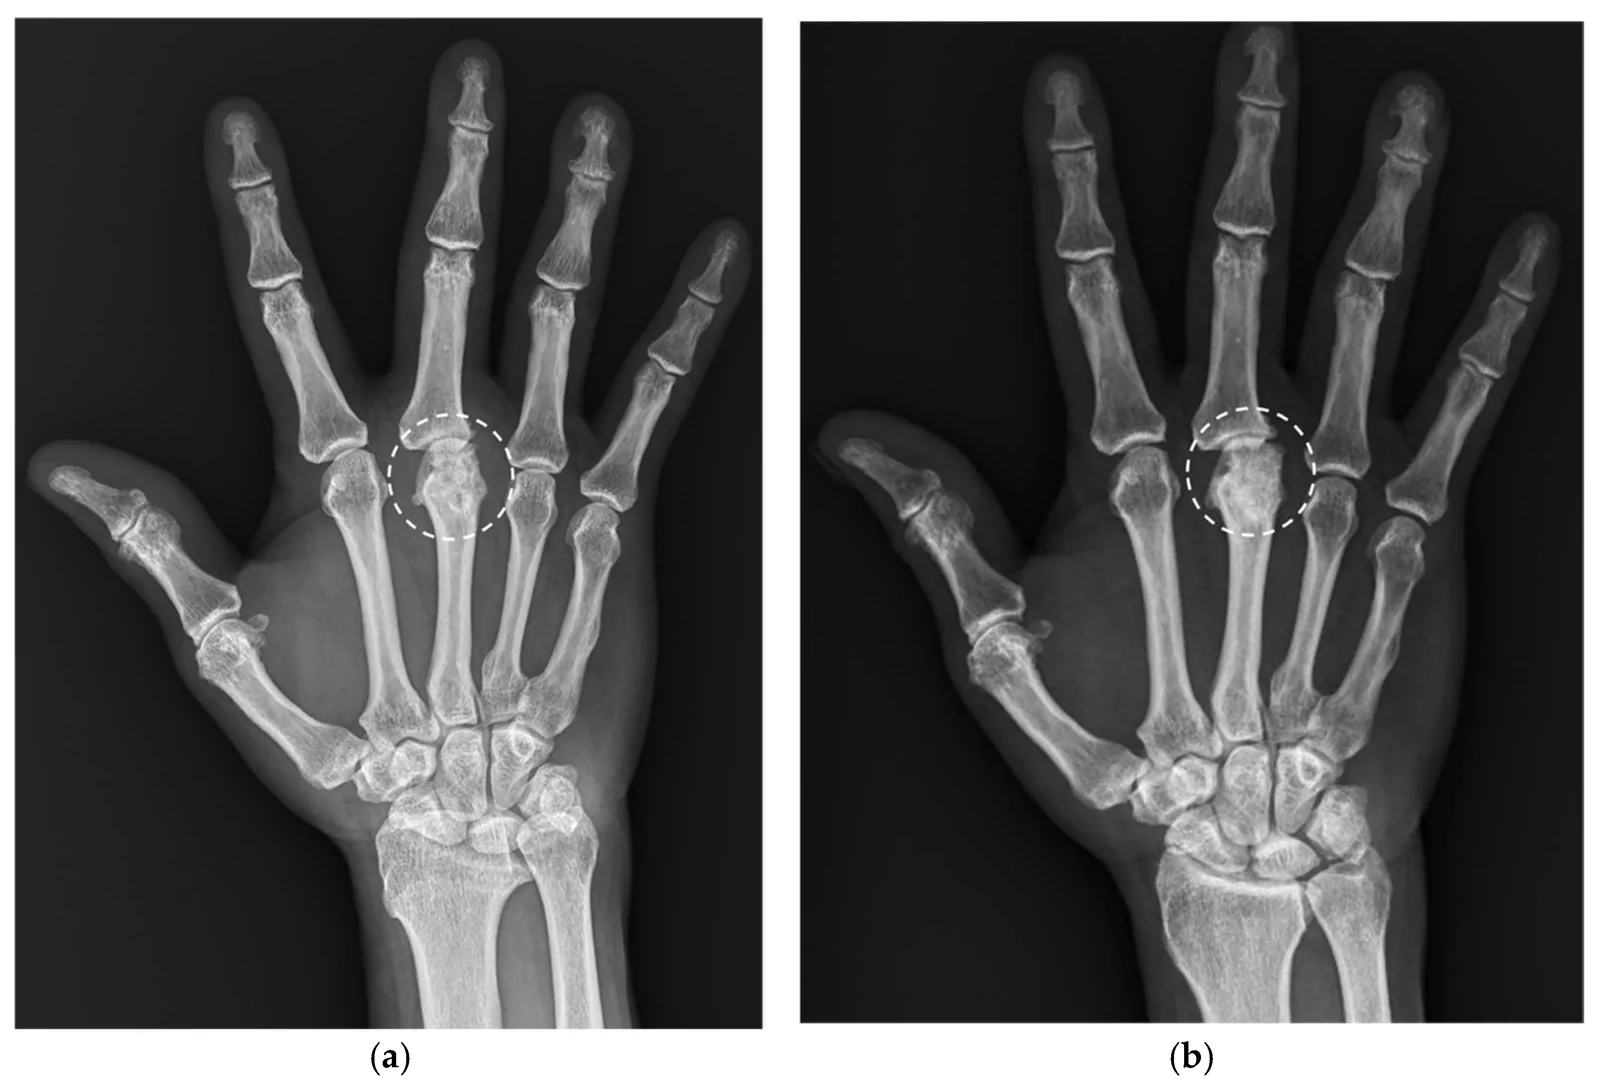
(a) Follow-up radiograph at postoperative 3 months showing progressive bone regeneration without collapse (dashed circle indicates the lesion). (b) Long-term follow-up radiograph at nearly 5 years (58 months) postoperatively showing complete bony integration of the autologous graft and progressive osseous regeneration of the third metacarpal head (dashed circle), without secondary collapse, articular degradation, or osteonecrotic recurrence.
